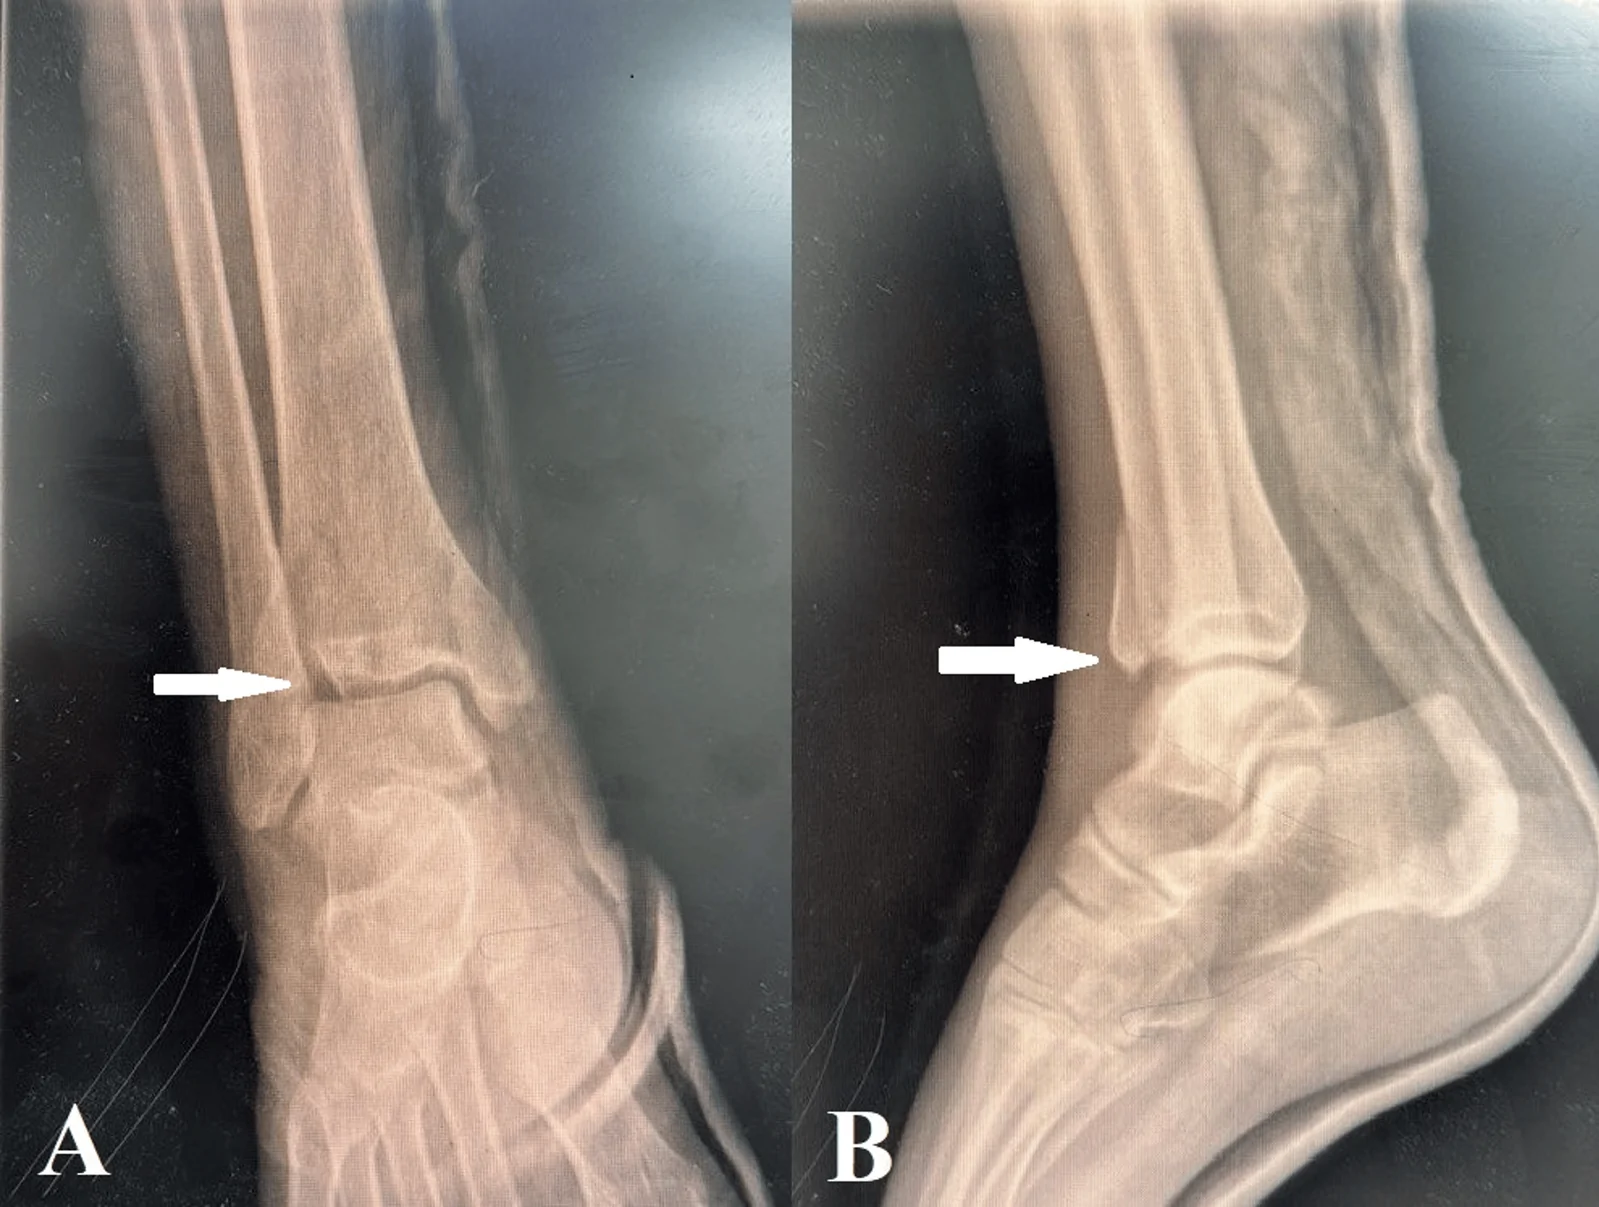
Initial X-rays. Initial presentation with anteroposterior (A) and lateral (B) X-ray views showing an isolated displaced Chaput fracture (arrow).
